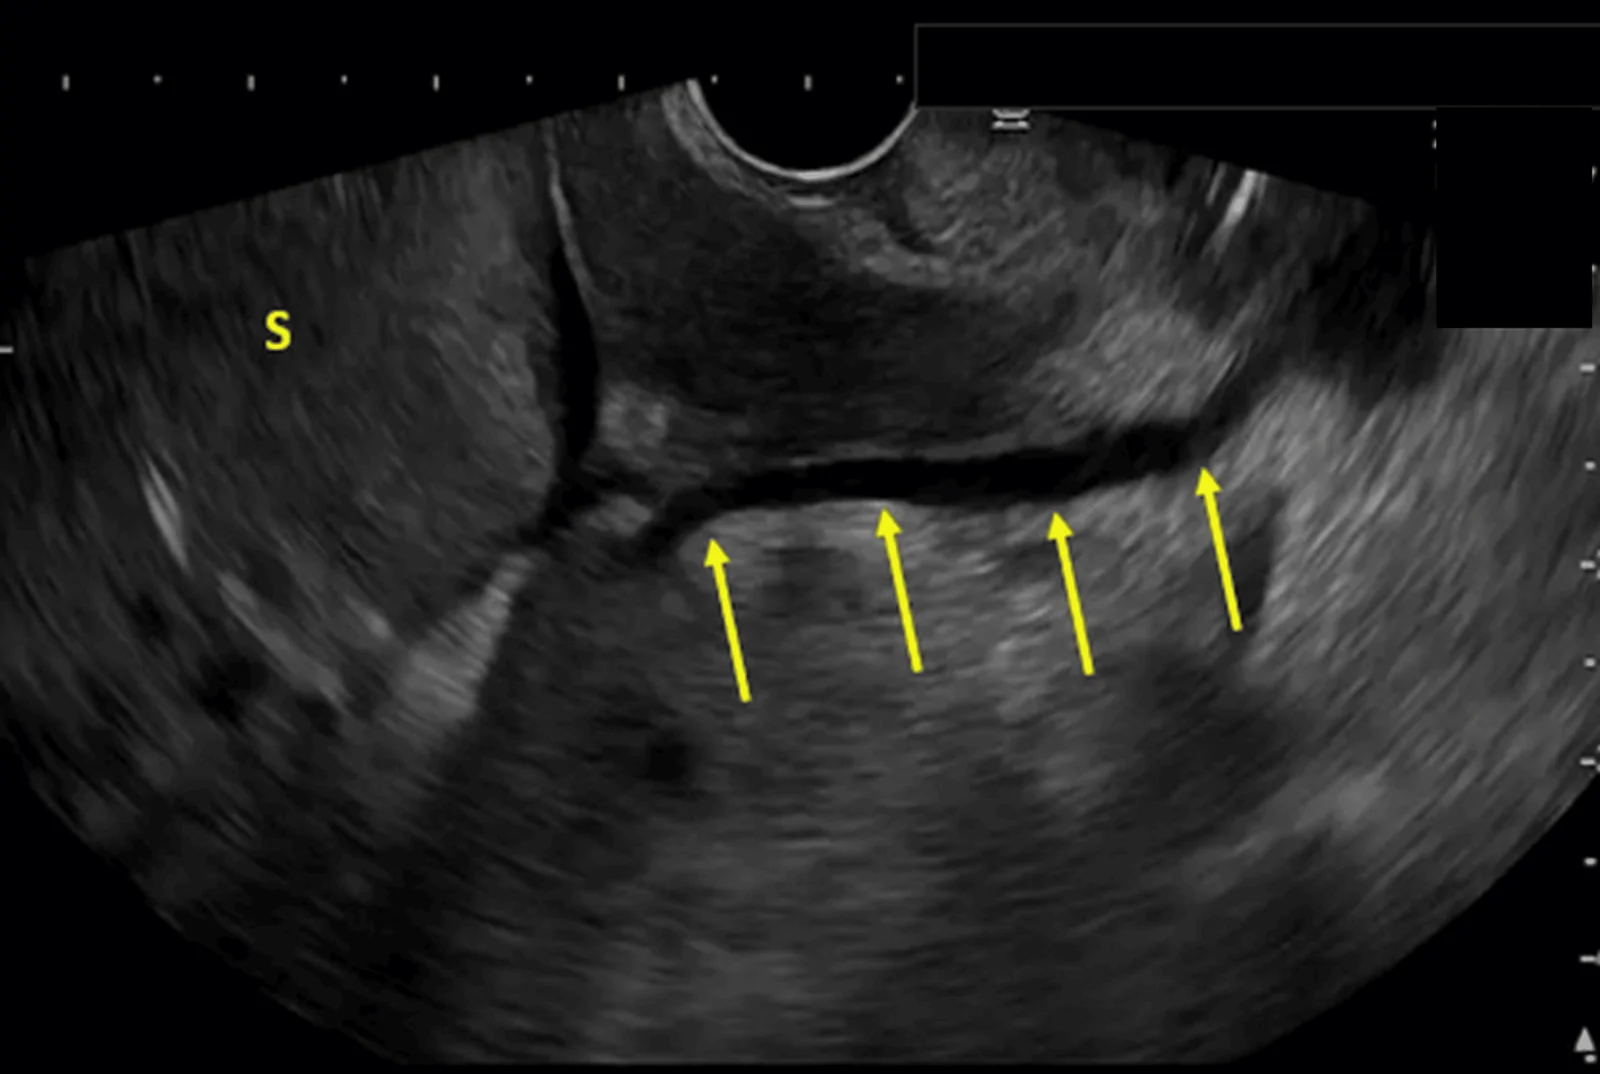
Radial endoscopic ultrasound (EUS) scan highlighting a narrow, elongated hypoechoic channel. The yellow arrows point to a markedly thickened pyloric muscle layer surrounding a compressed pyloric canal. ‘S’ is the stomach.
